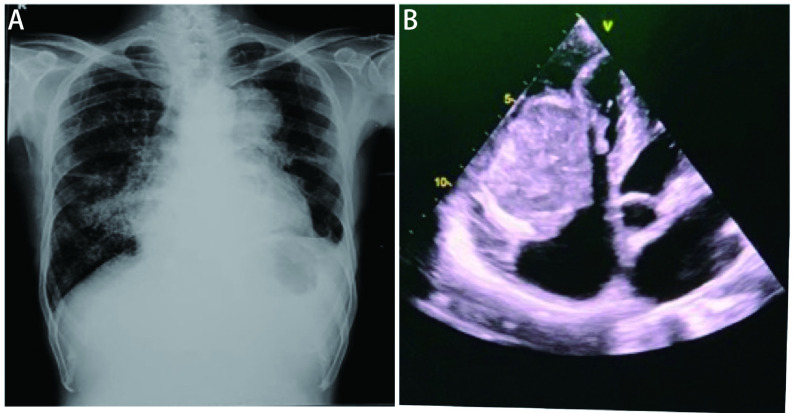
CXR and ultrasound images. A: CXR shows left upper zone homogenous opacity with left CP angle blunting and left diaphragm elevation, nodular opacities are seen in right middle and lower zone, cardiomegaly with prominent right cardiac borders and right fissure thickening; B: A mixed echoic mass measuring 4 cm×6 cm in RV in flow just below the tricuspid valve without causing significant turbulence across tricuspid valve. CXR: chest radiograph.
